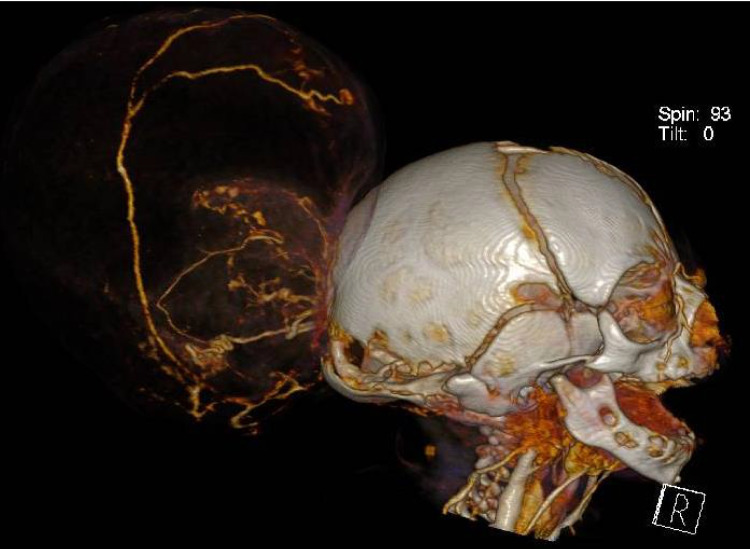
Preoperative CT-angiography
